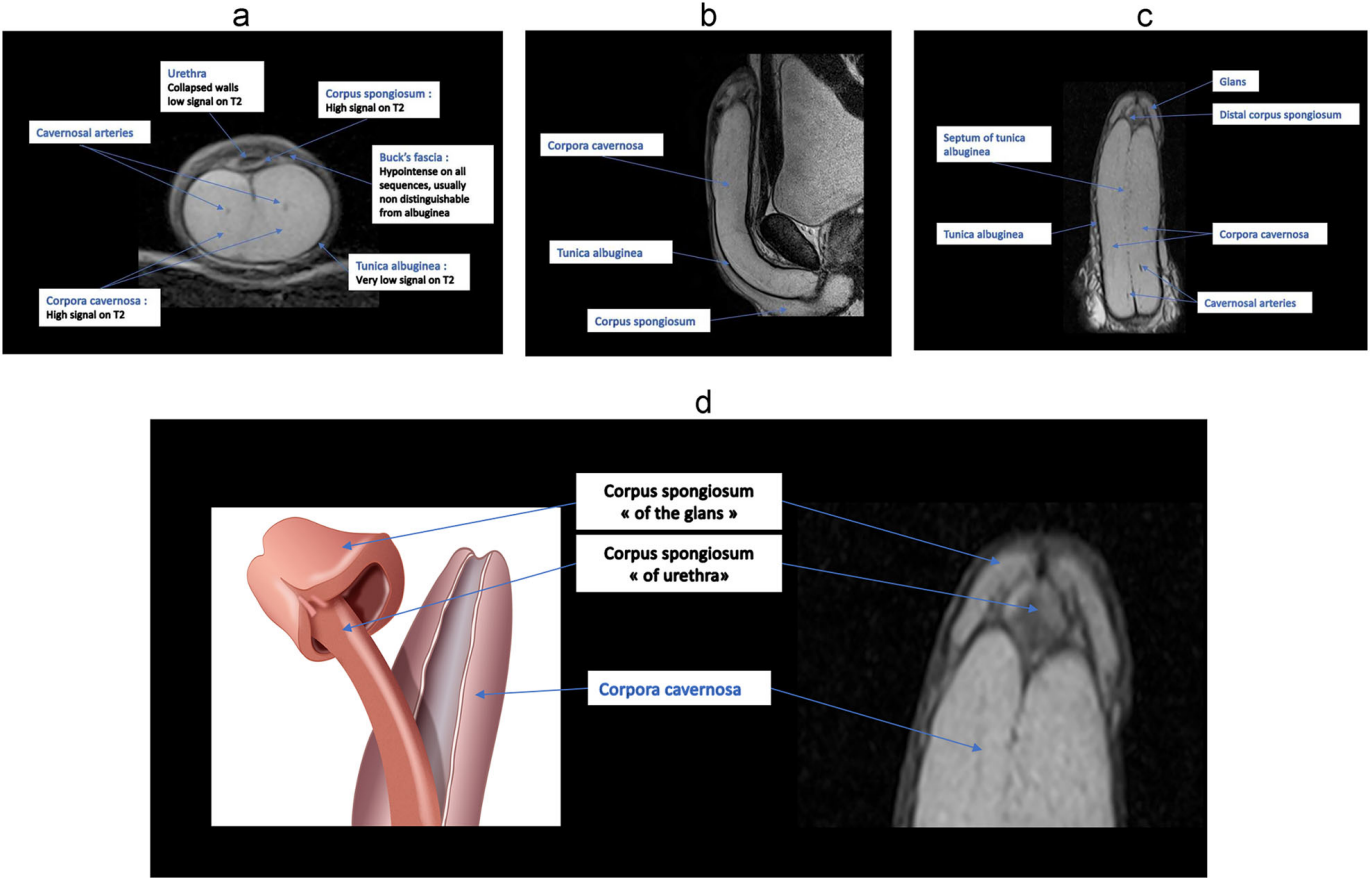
Normal penile anatomy using MRI. T2-weighted images, respectively, on an axial view (a), sagittal view (b), coronal view (c), and coronal view focused on the glans part (d)
